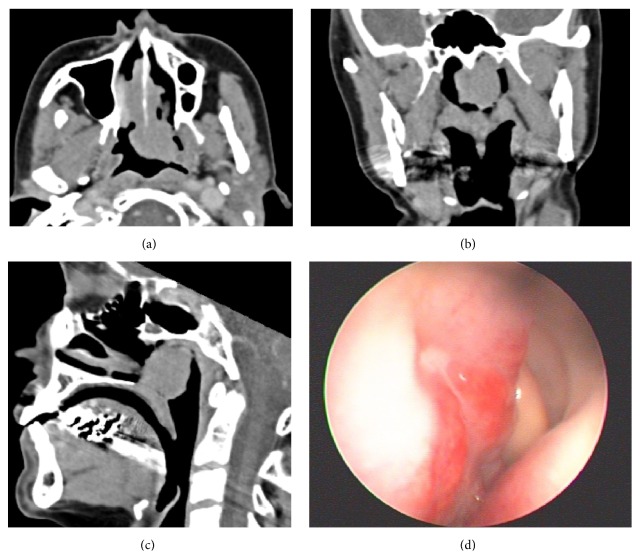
Imaging showing nasal septal lesion. CT images: (a) axial, (b) coronal, and (c) sagittal view. Endoscopic view left side: (d) lesion arising from the posterior part of the left nasal septum.
